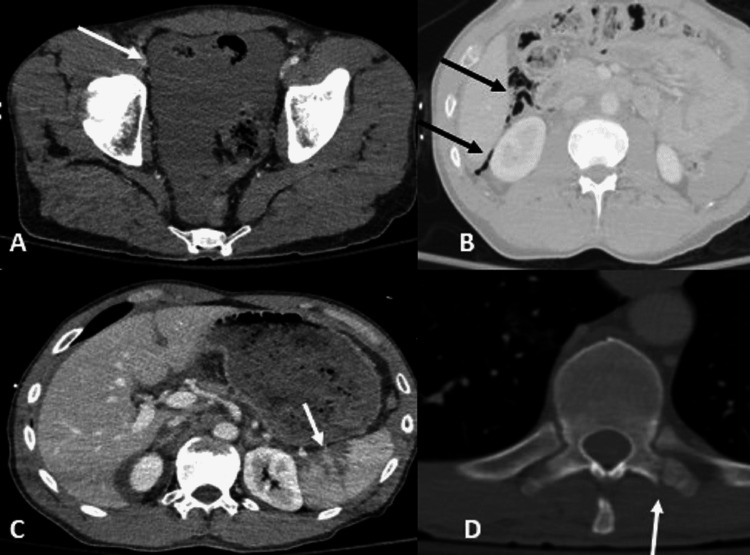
Postcontrast CT shows a large amount of free fluid in the abdomen and pelvis (A, white arrow), free air in right upper abdomen (B, black arrows), Splenic laceration (C, white arrow), and fracture of the left T10 transverse process (D, white arrow). A and C: CT with soft tissue window, B: CT with lung window, and D: CT with bone window.
